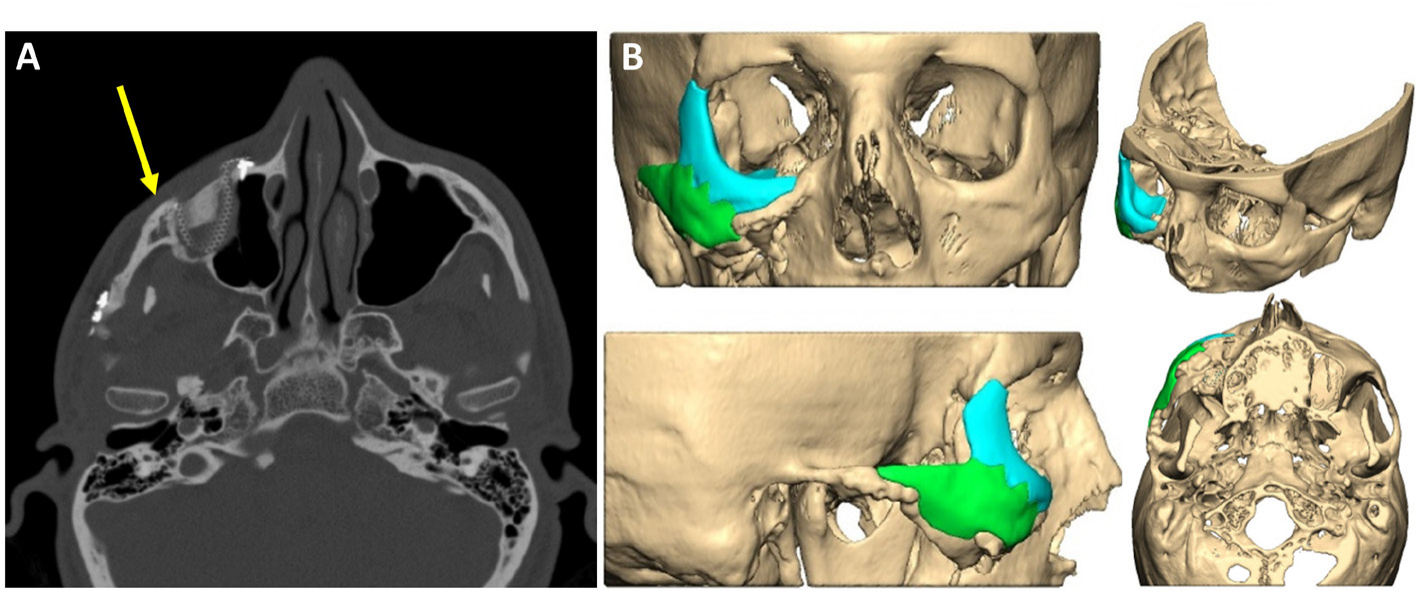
Patient-specific Implant for Reconstructing the Zygomatico-orbital Complex A: Axial CT reconstruction showing a patient suffering from improper reduction of fractures in the zygomatico-orbital complex. Yellow arrow shows the lacking projection of the malar eminence on the right side. B: A patient-specific implant made of polyether ether ketone (PEEK) was designed.
